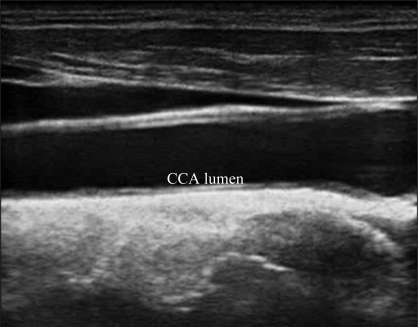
A sub-image from a B-mode sonographic image sequence. This patient has thin IMT. (Sequence S2: Male, 56 yr old).
